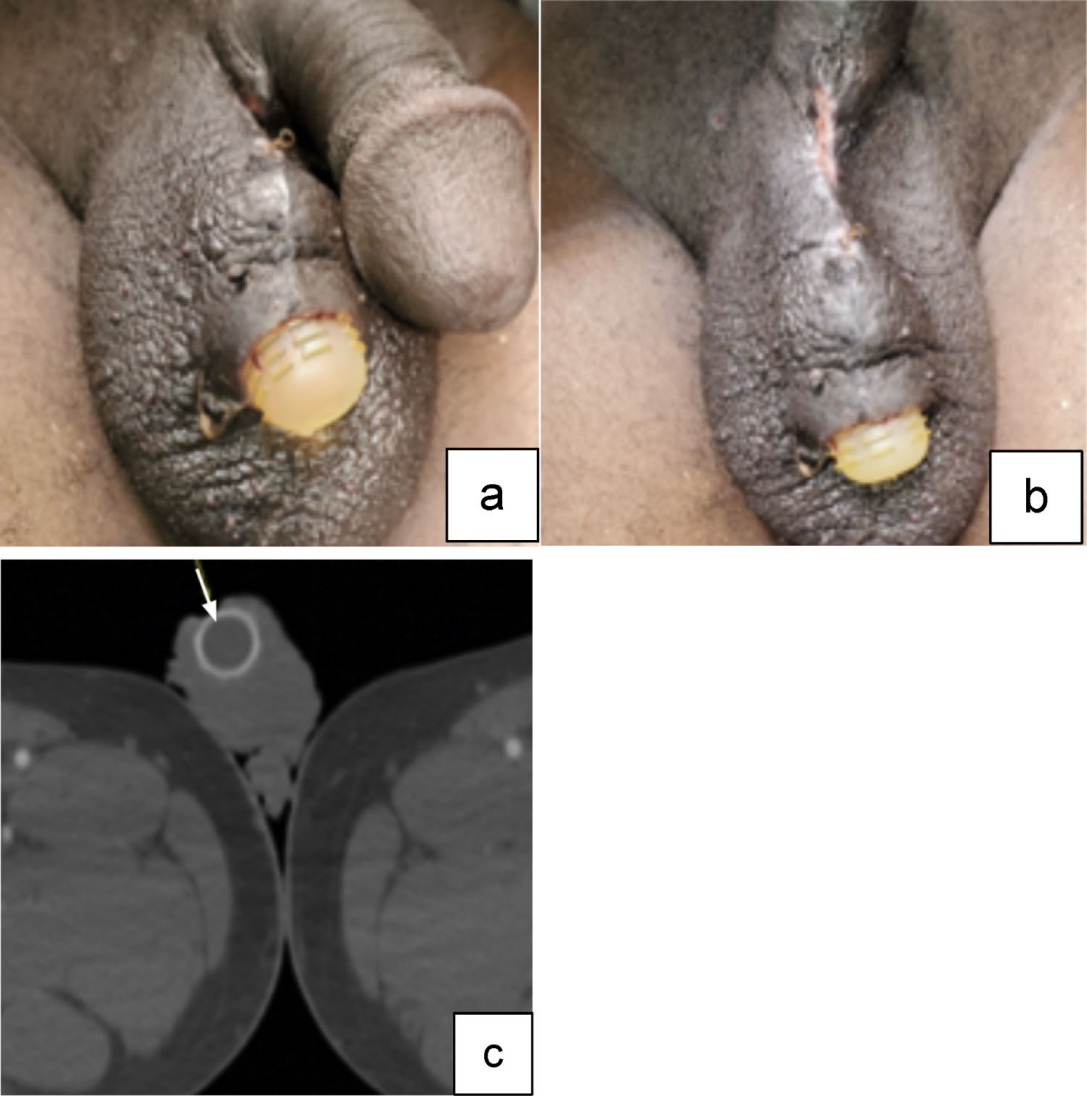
Photos show skin breakdown with the penile pump prosthesis protruding through the skin of the scrotum (a, b). Axial CT image with IV contrast (c) shows absence of skin barrier overlying the pump (white arrow)
